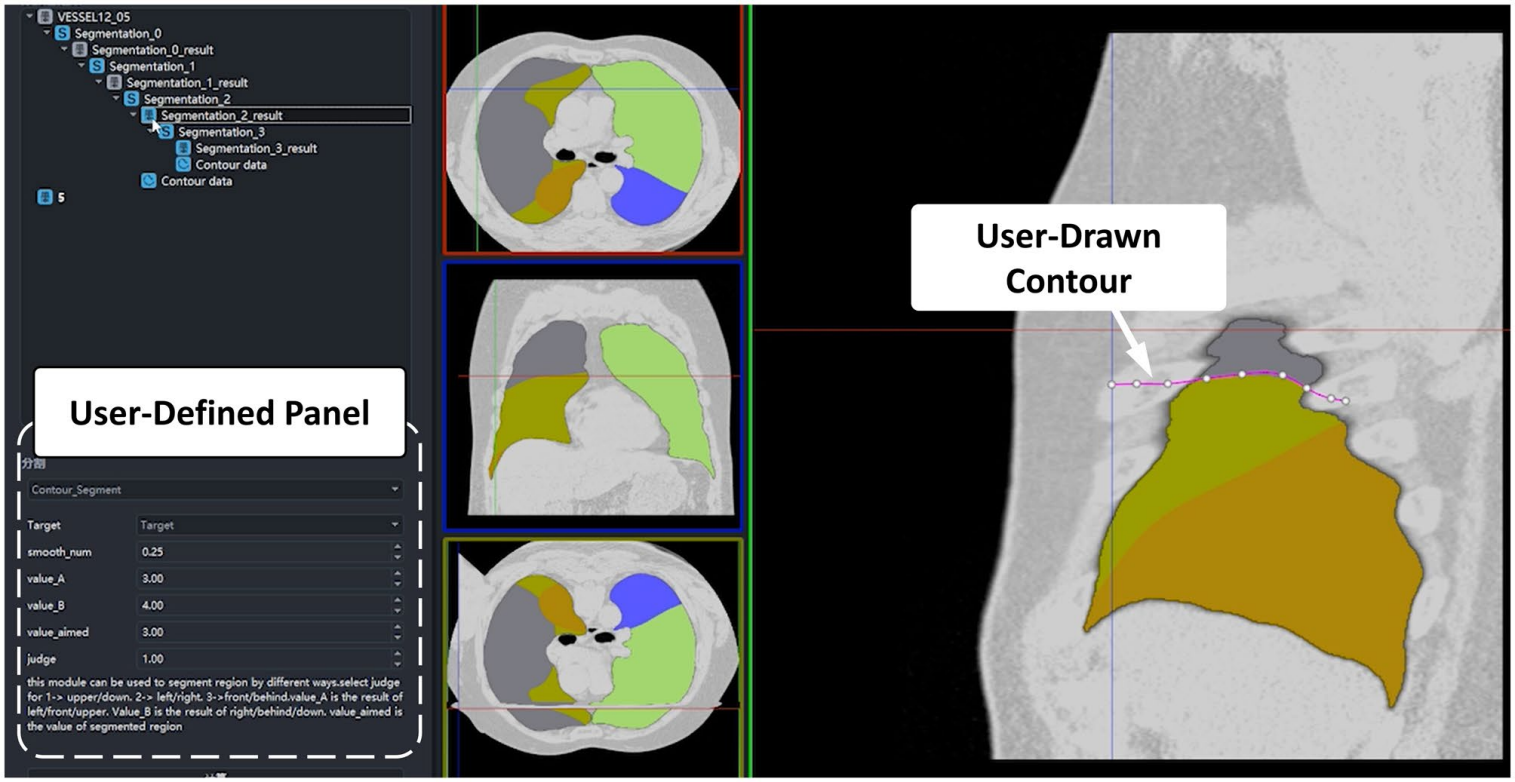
An example of user-developed plugin module for lung lobe annotation in CT images
